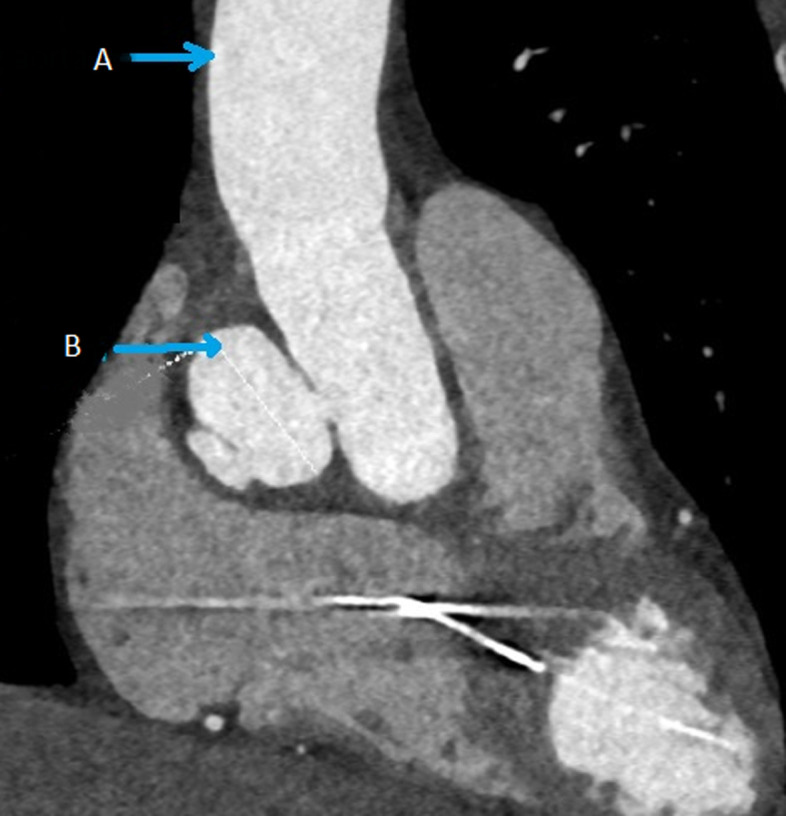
pre-operative computed tomography angiography scan showing prostheto-prosthetic pseudoaneurysm: A) ascending aorta; B) 3.3 cm pseudo aneurysm
